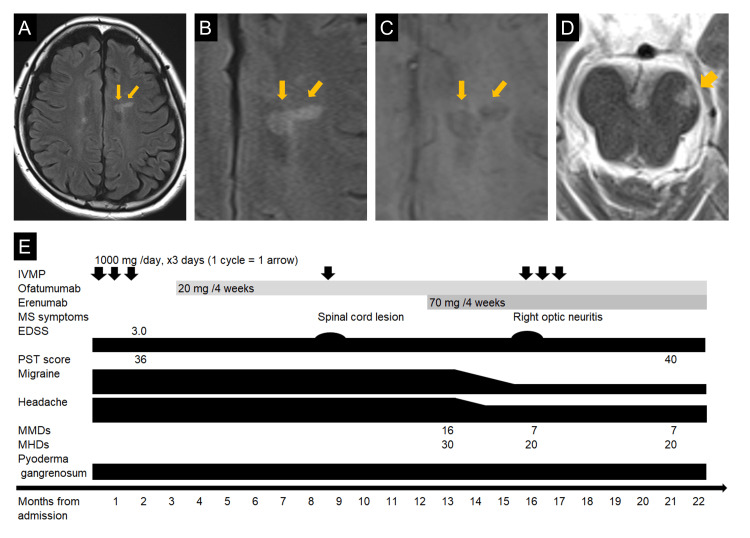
Brain MRI and Clinical Course An MRI examination of the patient, a 43-year-old woman, revealed a periventricular lesion and a paramagnetic rim lesion (indicated by arrows). The findings were observed on fluid-attenuated inversion recovery (A and B) and susceptibility-weighted imaging (C). A lesion involving the left cerebral peduncle was also identified (D). Throughout the clinical course, the patient's Expanded Disability Status Scale (EDSS) score and processing speed test (PST) score (E) did not deteriorate, and the number of her monthly migraine days (MMDs) and monthly headache days (MHDs) decreased IVMP, intravenous methylprednisolone; MS, multiple sclerosis
